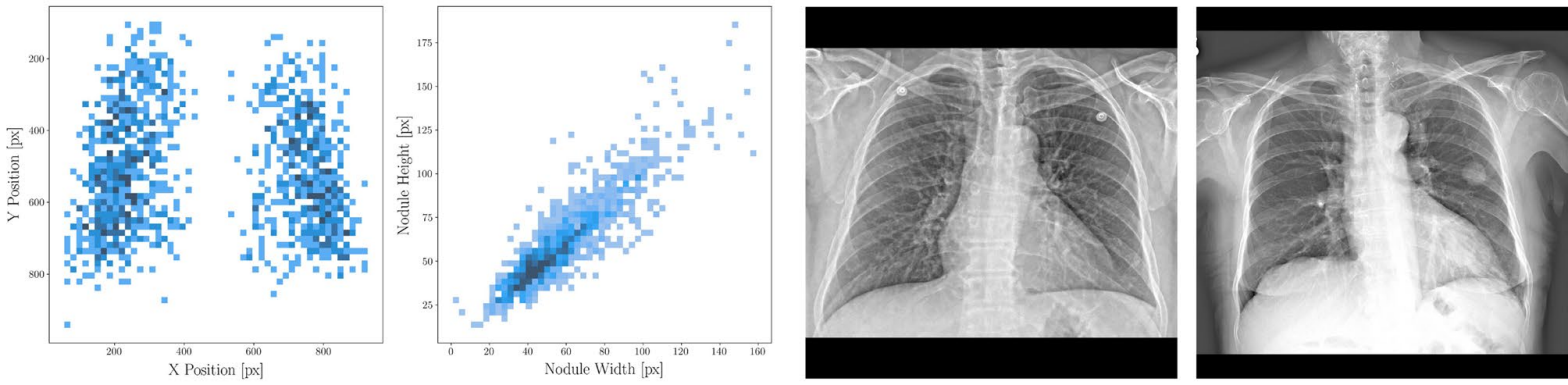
Analysis of bounding boxes in the data. From left to right: Position of all bounding boxes on the x and y axis height plotted against the width of all bounding boxes, respectively, exemplary x-ray without nodules, exemplary x-ray with one nodule.
